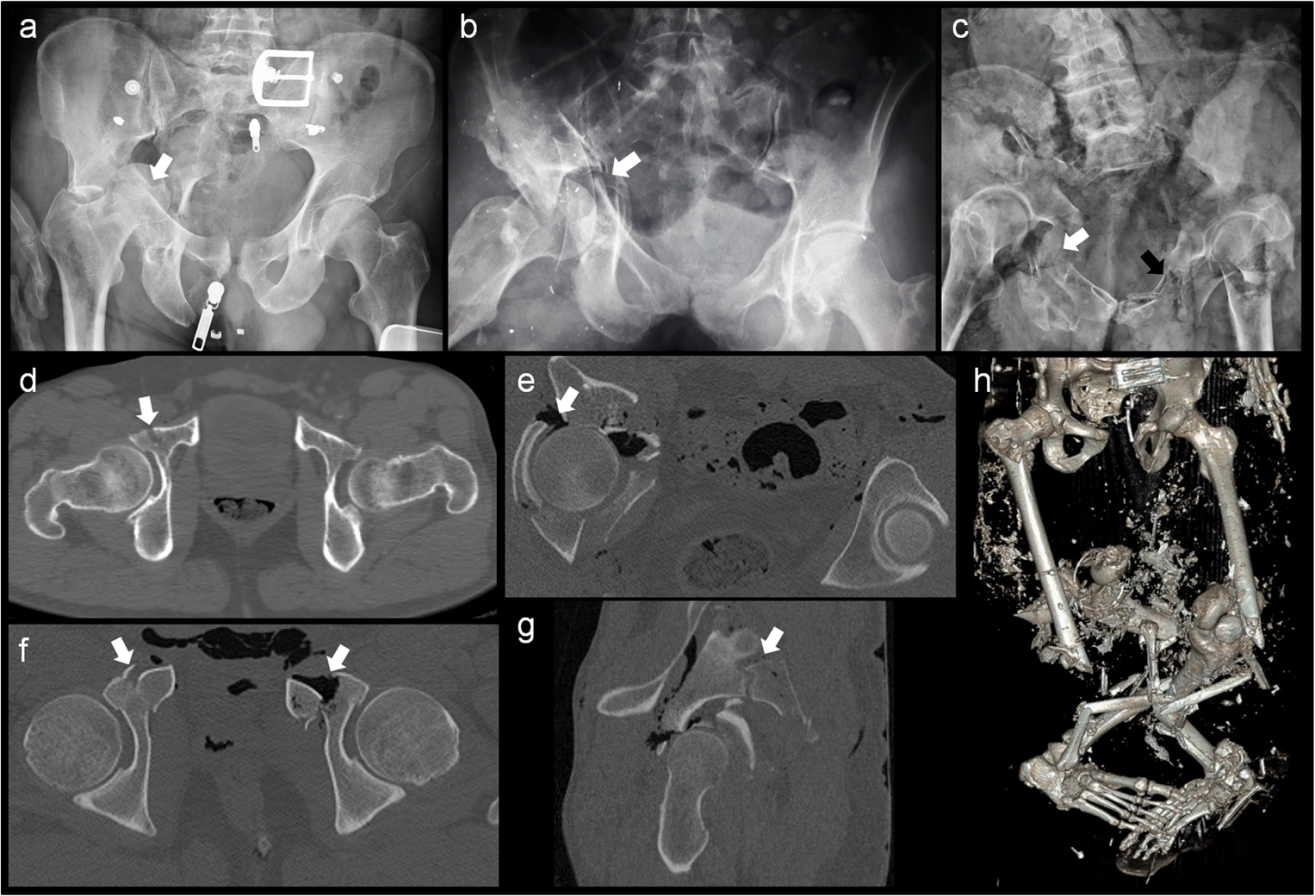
Examples of radiological images of acetabular fractures a Conventional radiographic anterior-posterior (AP) view of the pelvis. Right-sided acetabular fracture with medial directed penetration of the femoral head through the acetabulum (white arrow). b Conventional radiographic AP view of the pelvis. Both anterior and posterior column were fractured on the right side (white arrow). c Conventional radiographic AP view of the pelvis. Bilateral extensive fracture of both anterior and posterior columns (white and black arrows). d Transversal CT view of a subtle right-sided anterior column fracture (white arrow). e Transversal CT view of the pelvis. Right-sided comminuted anatomic acetabular fracture with penetration of the femoral head through the acetabulum (white arrow). f CT transversal view of a bilateral anterior column fracture (white arrows). g The same case as in f. Additional CT lateral view of the right hemi pelvis with comminution of the acetabulum. Note the acetabular roof fracture (white arrow). h 3D–CT reconstruction of the pelvis and lower extremities. The telescopic fracture pattern of the lower extremities is typical for aviation accidents [15]. Note also multiple pelvic fractures and diastasis of the symphysis in contrast to the seemingly intact pelvic region of the body (Fig. 4e)
